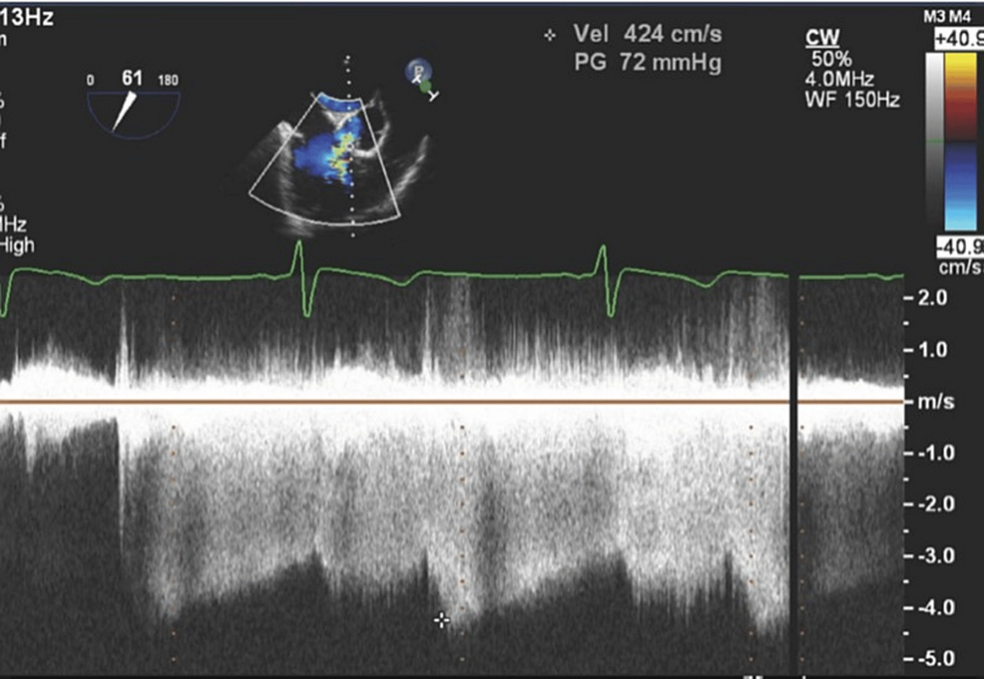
Transesophageal Echocardiogram (TEE). Mid-esophageal TEE still frame showing continuous doppler analysis of the left-to-right aorta-to-right ventricle shunt seen in SOVAR-C1-2. Doppler shows a continuous left-to-right shunt (below the line). A high pressure gradient (peak velocity over 4.2 m/s, consistent with a peak gradient across the defect grater of 72 mmHg) suggests normal right ventricular pressures.
